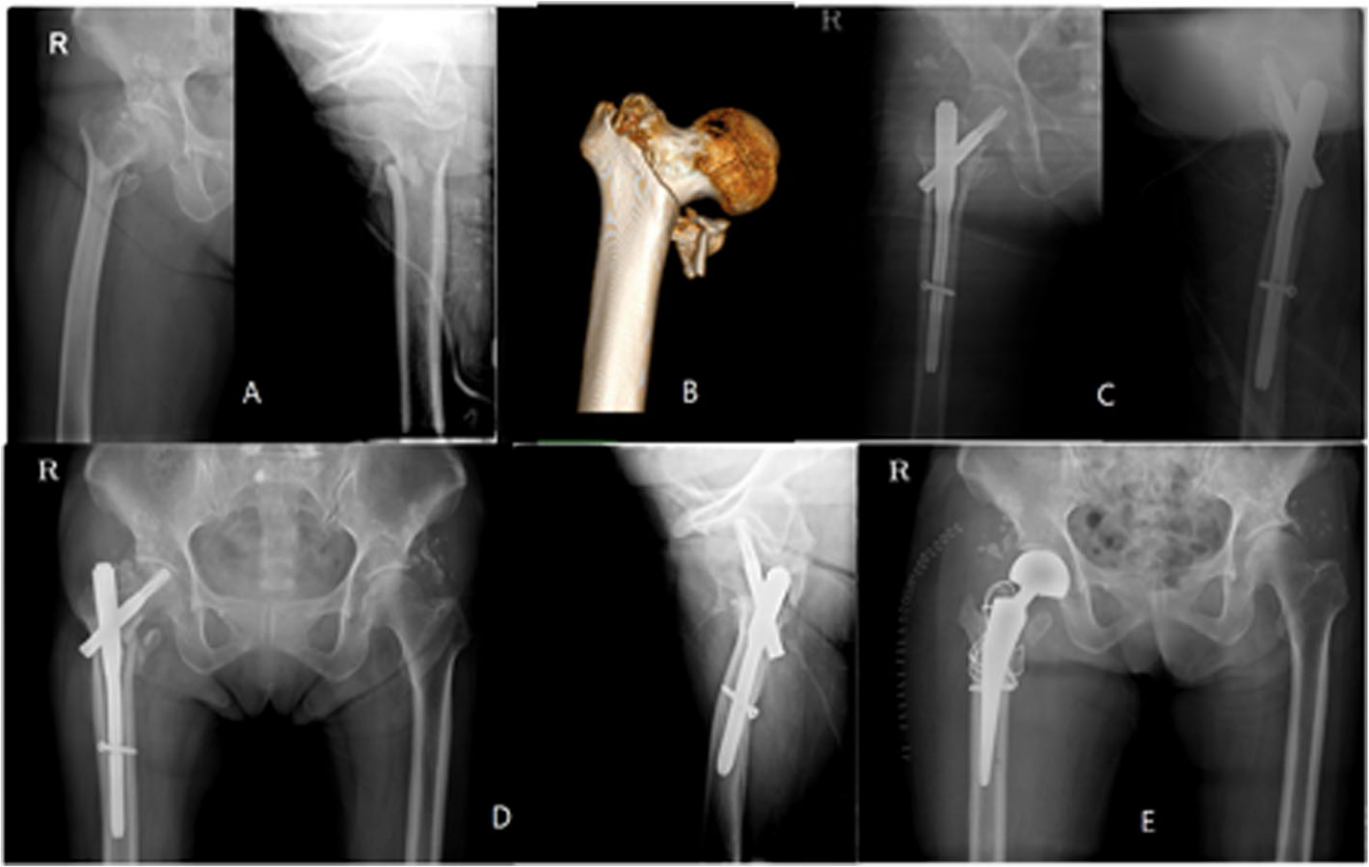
An 81-year-old woman fell on the ground; the proximal fragment was short with varus angulation (a). 3D-CT imaging showed a basilar neck fracture (b). The fragment was reduced using PFNA II screws (c). The lag screw demonstrated cut-through 3 months postoperatively (d). We converted it via hemiarthroplasty (e)
